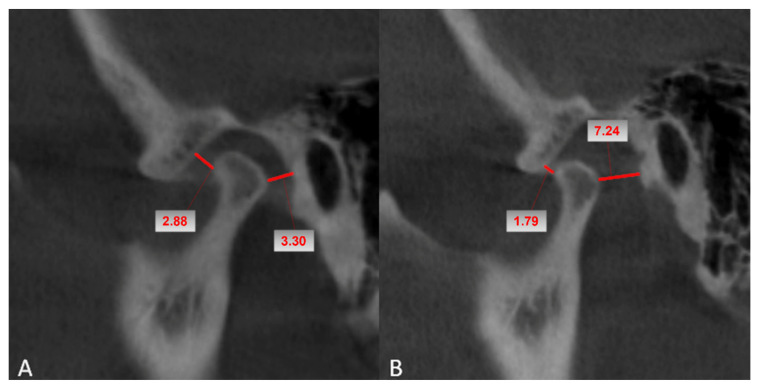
CBCT examination with a closed mouth. Sagittal plane. TMJ anterior and posterior space measurements: (A) preoperative, (B) postoperative.
